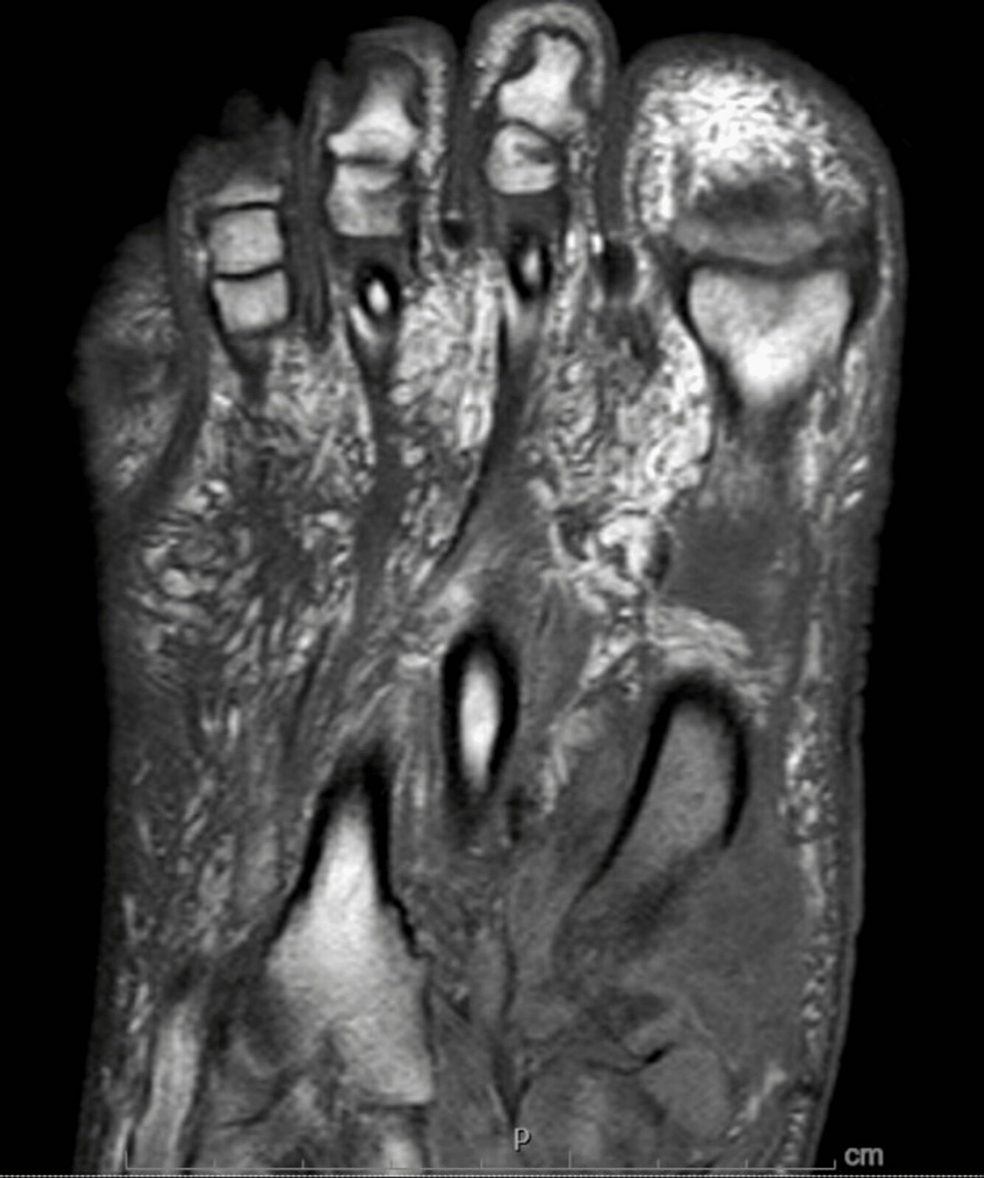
MRI right foot T1 coronal view with septic first tarsometatarsal joint with complex joint effusion, complex periarticular abscess measuring 35 mm, osteomyelitis of medial cuneiform and first metatarsal base with associated mildly displaced pathologic fracture of the first metatarsal base.
